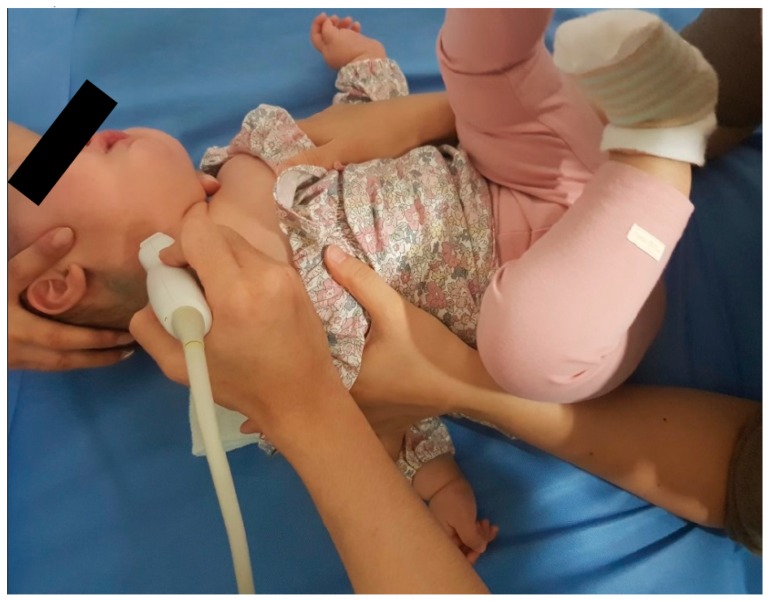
The position of the infant during ultrasonography to the sternocleidomastoid (SCM) muscle in the transverse plane.
